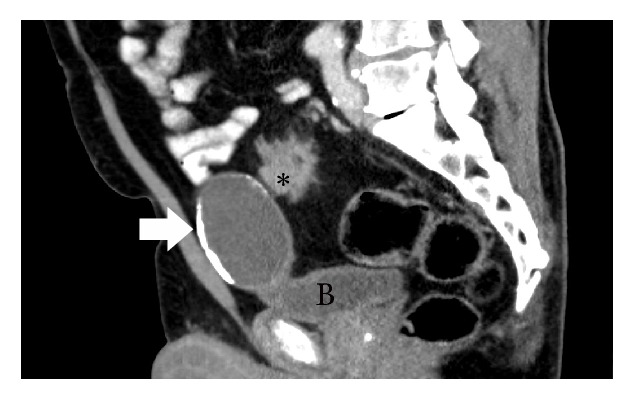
Sagittal image from contrast enhanced CT demonstrating a 6.9 cm rim calcified cyst (see Arrow) arising from the dome of the urinary bladder (labelled “B”) corresponding to a urachal cystic tumor of low malignant potential. Immediately posterior to this is the 6.5 cm sigmoid colon adenocarcinoma (labelled *∗*), represented as circumferentially thickened bowel with luminal narrowing and irregular serosal surface seen in cross-section.
